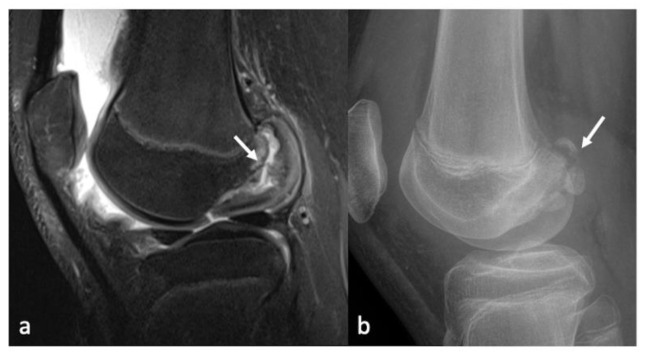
PDFS sagittal (a) and lateral radiographs of the knee (b) showing unstable OCD of the lateral femoral condyle (arrow).
